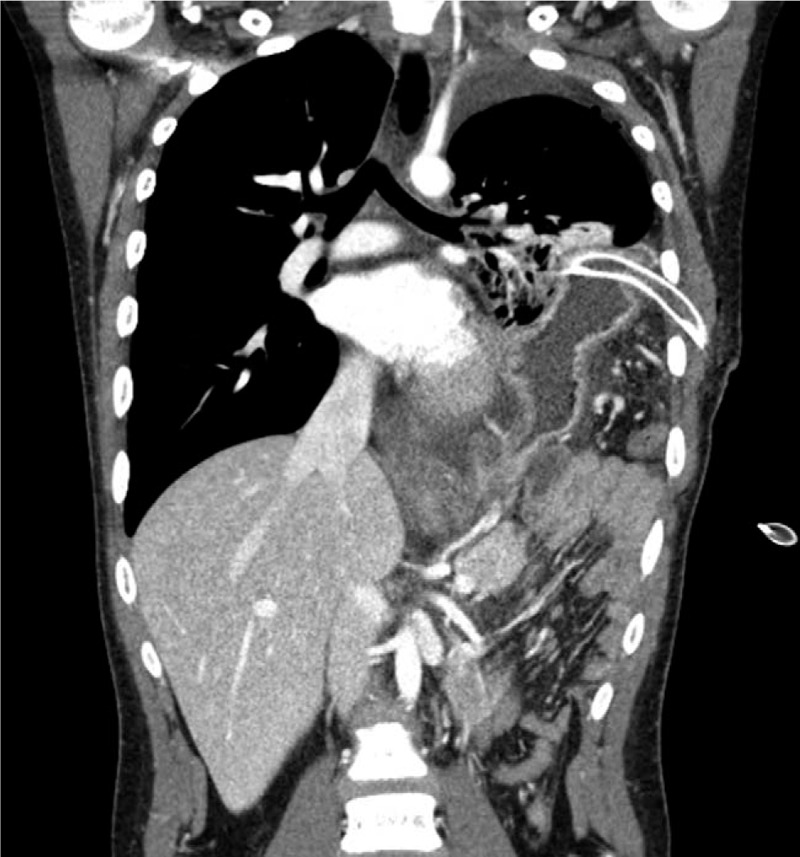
Chest computed tomography (CT) shows congenital diaphragmatic hernia (CDH) and iatrogenic gastric perforation caused by chest tube thoracotomy.
